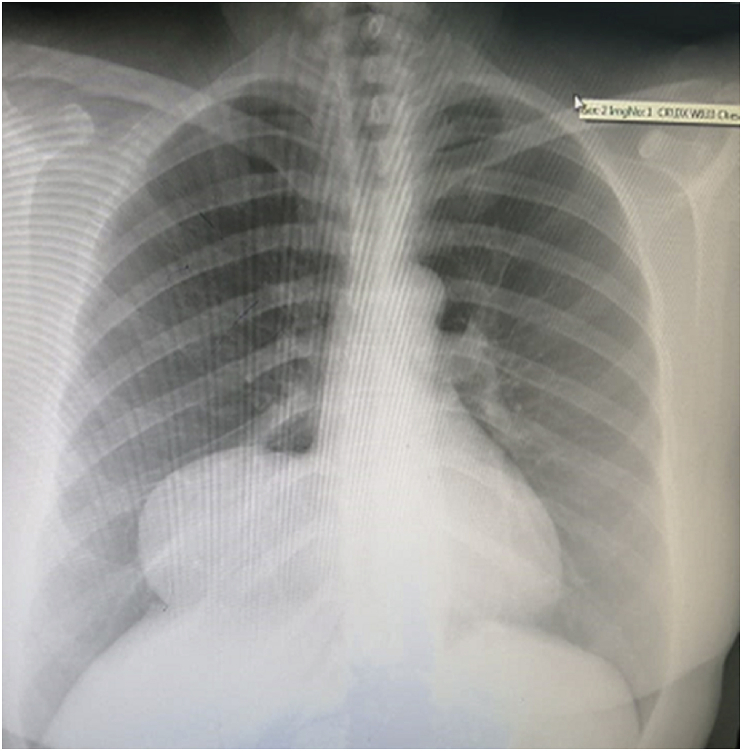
A chest x-ray shows a well-defined oval like opacification at the right cardiophrenic angle.
